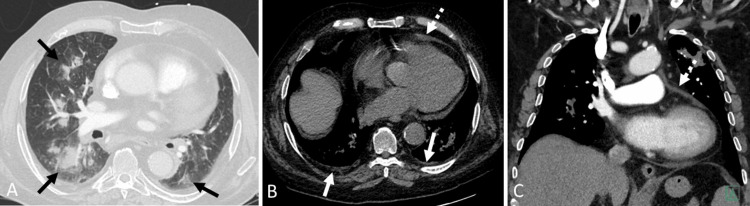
Chest CT (A) Axial lung window image reveals patchy consolidative opacities with areas of ground-glass attenuation in both lungs, consistent with neutrophilic alveolitis (black arrows). (B) Axial and (C) coronal soft tissue window images show mild pericardial effusion (dashed white arrow) and trace bilateral pleural effusions (solid white arrow).
